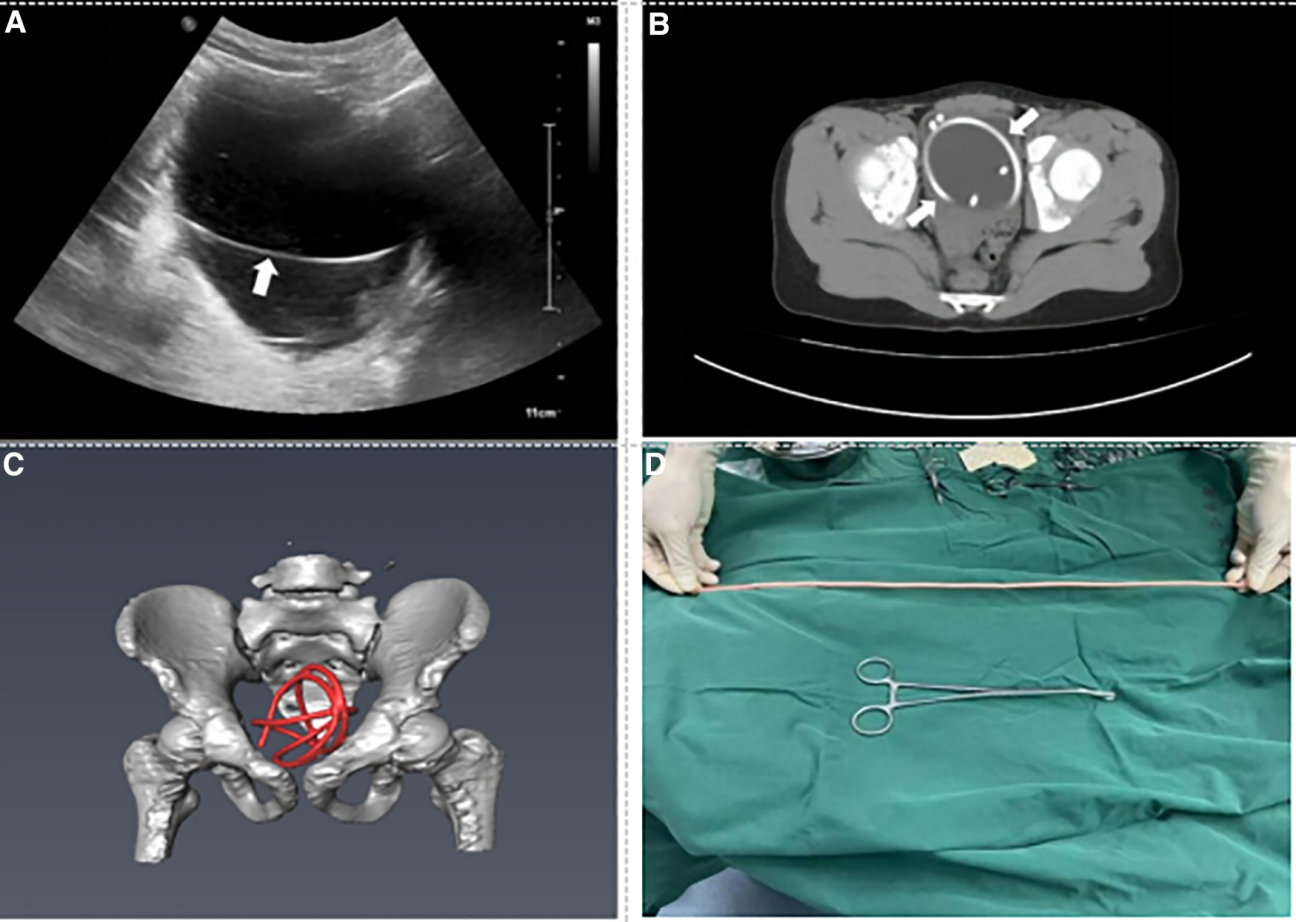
Preoperative imaging data and foreign body. (A) US shows the bladder foreign body (see arrow). (B) CT of the pelvis shows the crooked foreign body in the bladder (see arrow). (C) Three-dimensional reconstruction CT shows the lumpy foreign body in the bladder (red mark). (D) Foreign body is a skipping rope about 50 cm long.
